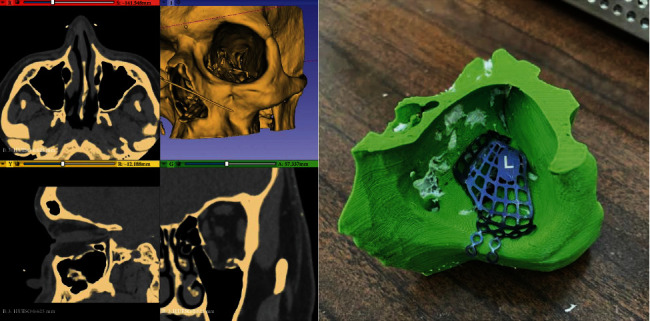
Computerized tomography (CT) of coronal, transverse, and sagittal planes that shows the orbital floor fracture. 3D-printed CT model is shown with the appropriate mesh, and this technique is used to provide a better approach in the treatment of skull fractures. Used with permission from the author Dr. Javier Asensio-Salazar.
